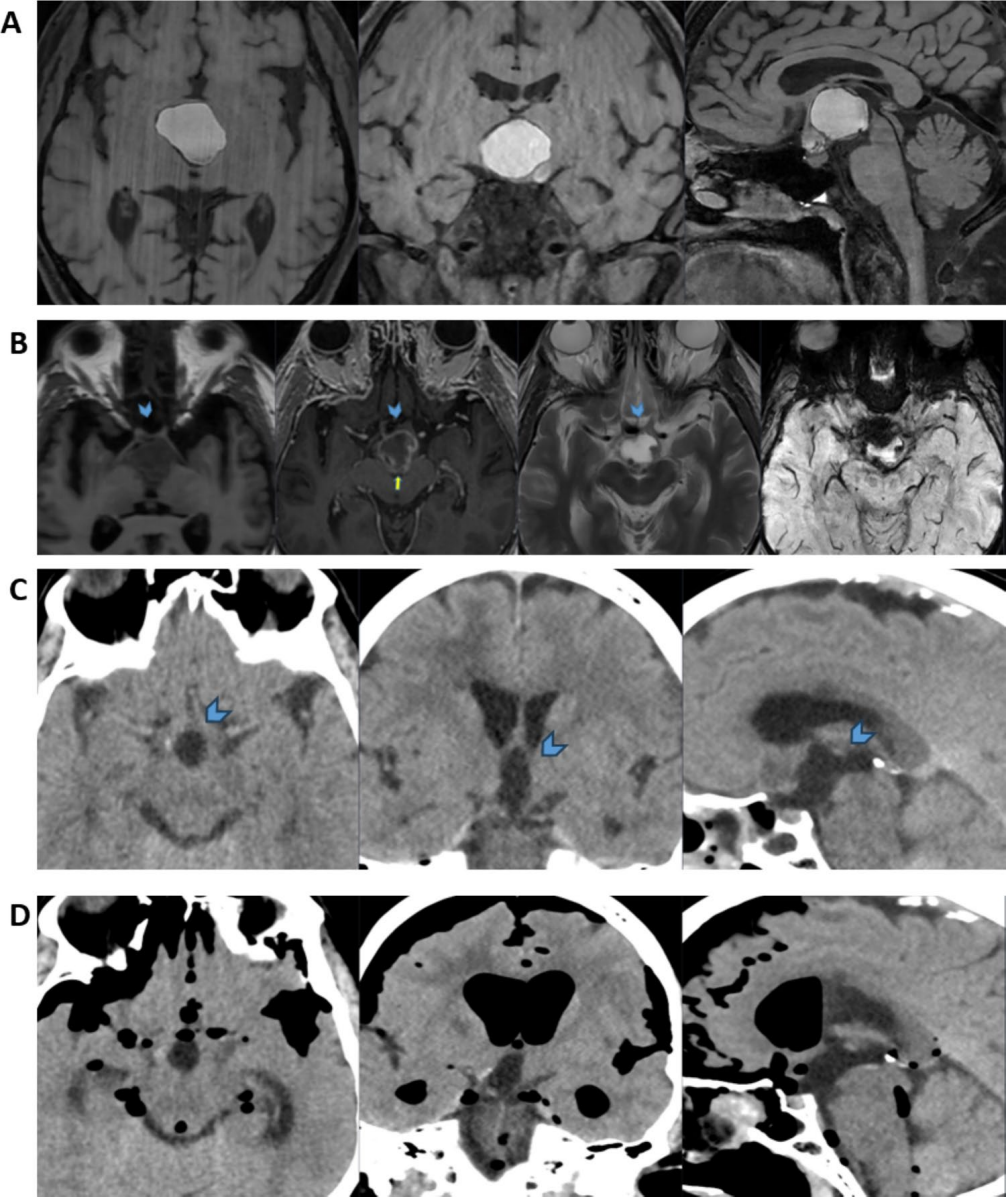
A) Native T1-w, axial, coronal, and sagittal views. Large predominantly cystic suprasellar expansiveness. The lesion is located in the suprasellar cistern, as appreciated in the sagittal view. Native high signal on T1-w is seen, suggestive of a significant intra-lesional subacute bleeding. B) Axial native T1-w, CE T1-w, T2-w, and SWI. MRI post endoscopic fenestration of cystic craniopharyngioma, via a right frontal approach. Compared to the preoperative MRI dated 2023–08–09, a centimeter-sized reduction of the cyst in all axes is appreciated. Slight edema and minor amounts of postoperative blood and small amounts of intraventricular air. The contrast enhancement in the cyst wall was seen as slightly increased compared to previous imaging (yellow arrow), and interpreted as postoperative-related changes. The optic chiasm is located anteriorly and is elevated by the cyst (blue arrowhead). C) Axial, coronal, and sagittal brain CT, soft-tissue window. Post resection status, no evident residual tumor, and no major bleeding (blue arrowhead). D) Onset of extensive supra- and infratentorial pneumocephalus, including intraventricular air causing hydrocephalus. High-attenuating hematoma posteriorly in the ethmoidal sinus (yellow arrow).
